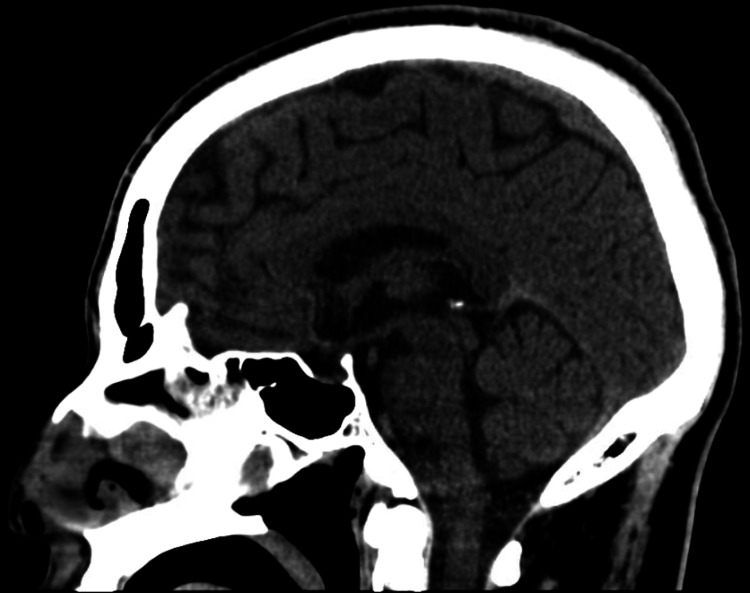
CT head showing normal findings
